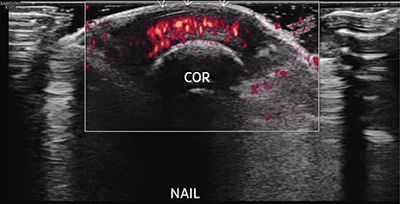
Transverse ultrasound shows increased subungeal power Doppler signal.
